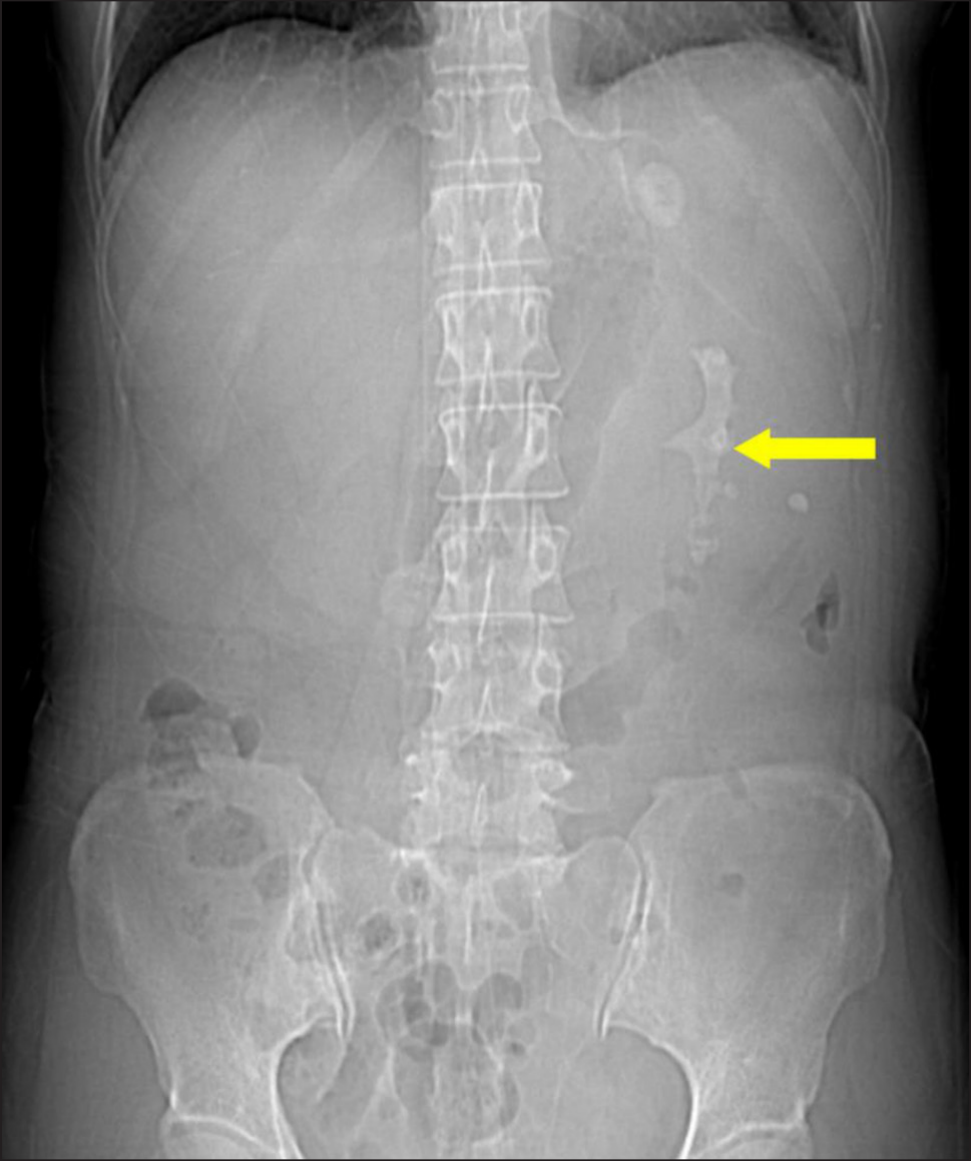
CT scanogram shows a staghorn calculus (arrow) in the location of the left kidney.
